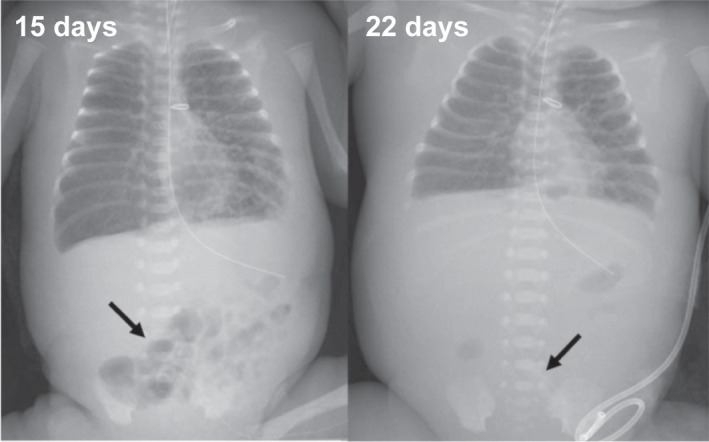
PICC tip position on the radiograph. The arrow points to the tip of the PICC from the left great saphenous vein on the 15^th^ and 22^nd^ day
